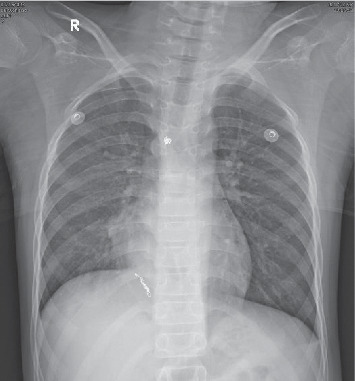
One day after bronchoscopy, no evidence of atelectasis was found on the chest X-ray.
